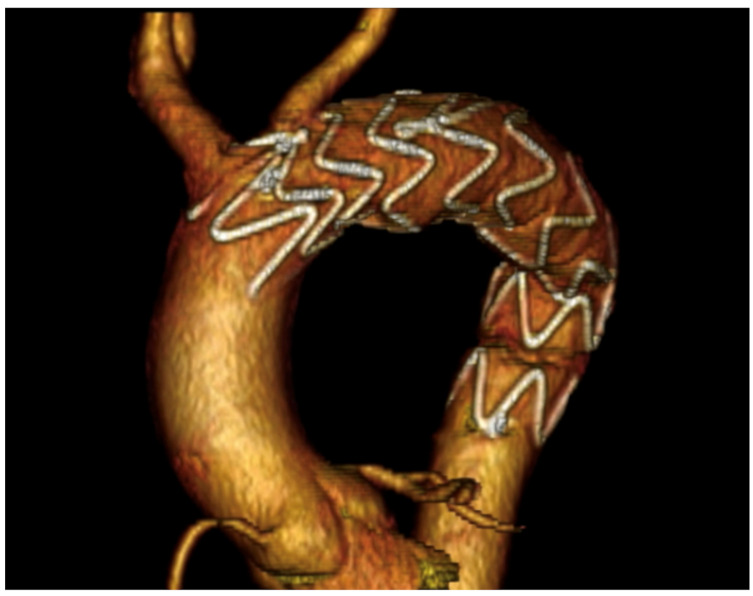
Three-dimensional volume-rendered reconstruction of the post-operative 4-year CTA.
